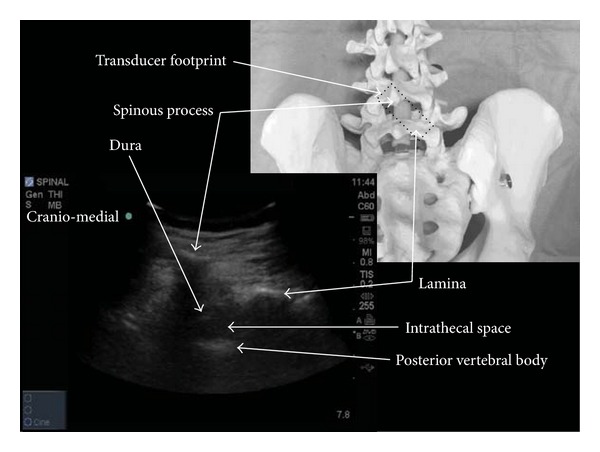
Spine model showing the paramedian oblique transducer orientation (rotated by 45 degrees) used during real-time scanning with a typical ultrasound image.
